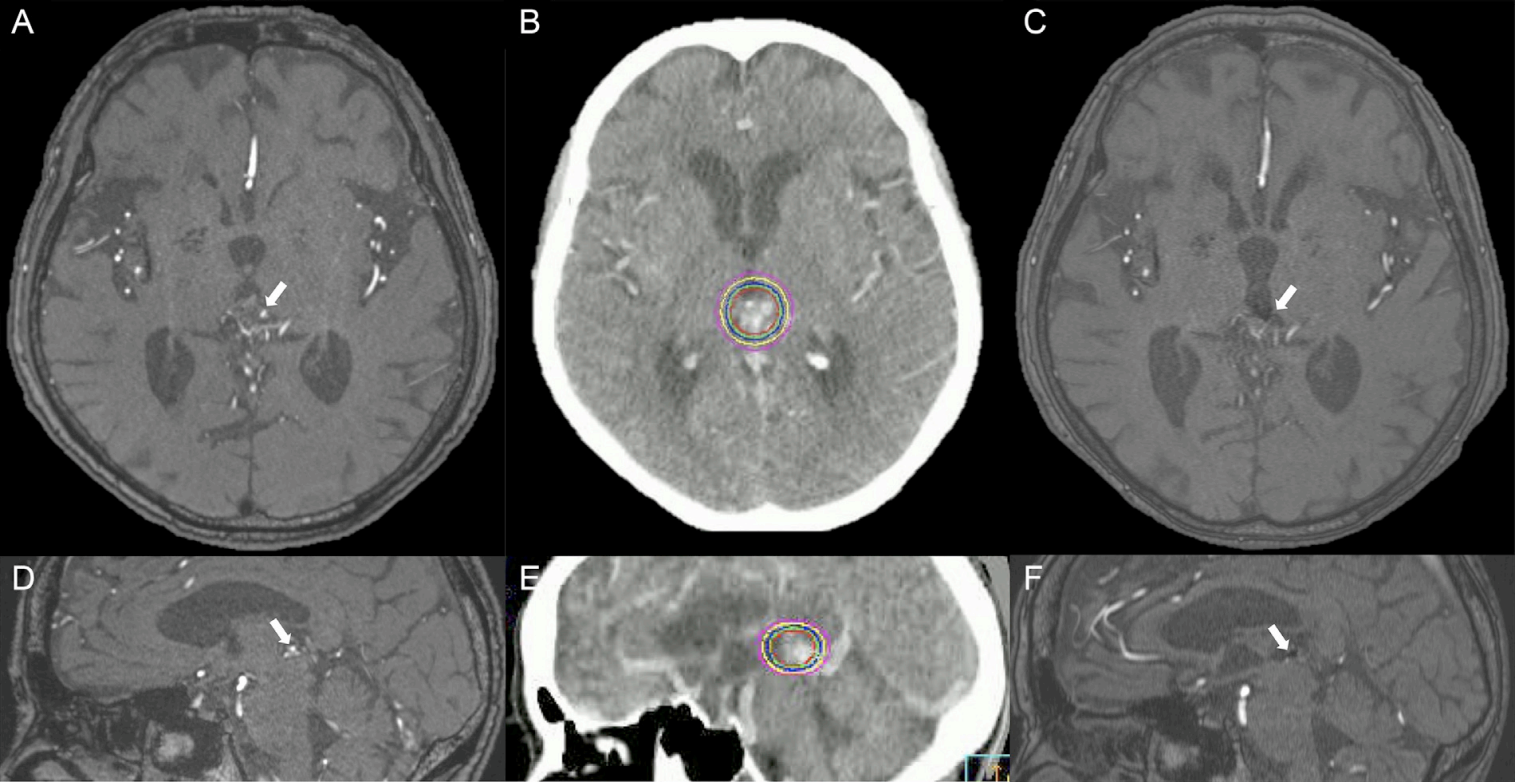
Axial (A) and sagittal (D) magnetic resonance angiography before linear accelerator-based stereotactic radiosurgery reveal an aneurysm (white arrow). Axial (B) and sagittal (E) computed tomography show the radiation field and dose color of radiation (pink circle: 12 Gy, yellow circle: 15 Gy, blue circle: 18 Gy, green circle: 19 Gy, red circle: 20 Gy). Axial (C) and sagittal (F) magnetic resonance angiography 4 months after linear accelerator-based stereotactic radiosurgery shows the disappearance of the aneurysm (white arrow).
